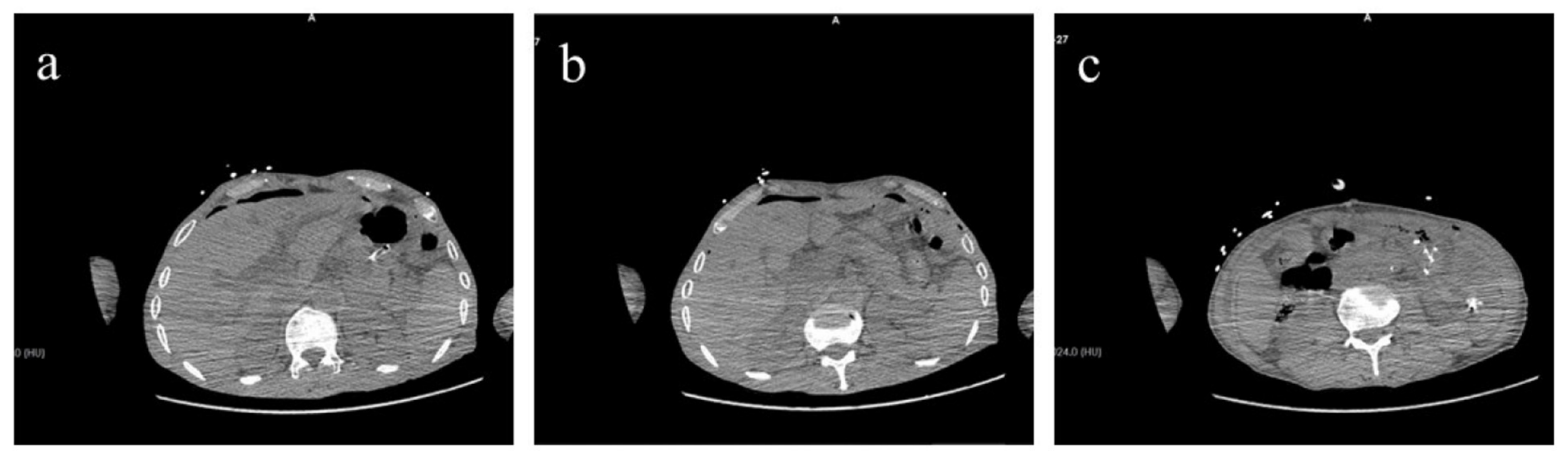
Postoperative imaging confirming resolution of obstruction. (a–c) Sequential axial CT slices demonstrate absence of small bowel air-fluid levels and normalization of the intestinal lumen, confirming successful surgical management of the obstruction.
